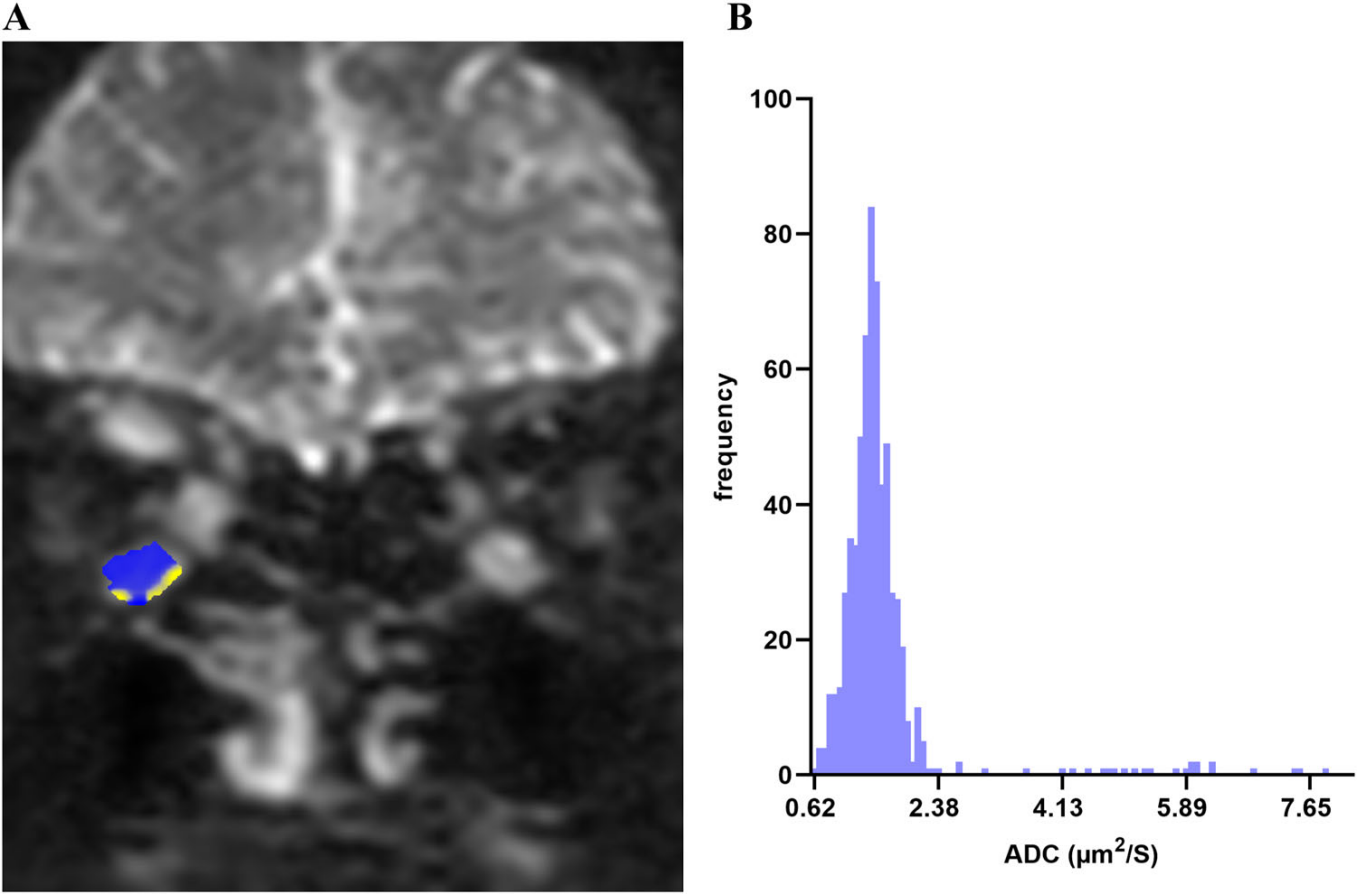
Patient with active TAO (male, 46 years). A. DWI coronal image with *b* = 0, showing bilateral inflammatory edema of the medial and inferior rectus muscles and the right superior rectus muscle, with markedly increased signal. The blue‐yellow area of the right inferior rectus muscle is the area of interest at this level. (B) Histogram of the ADC of the right inferior rectus muscle, reflecting the distribution of each ADC value. ADC, apparent diffusion coefficient; DWI, diffusion‐weighted imaging; TAO, thyroid‐associated ophthalmopathy.
